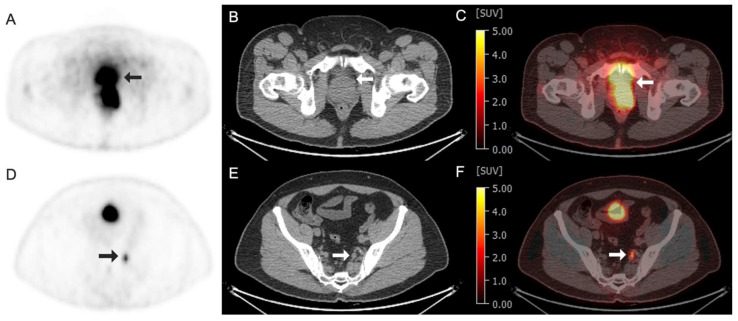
Images obtained from a 60-year-old man with prostate cancer at the level of prostate (A–C) and internal iliac node (D–F). (A–C) Arrows demonstrated an intense radiotracer uptake at the left aspect of the prostate, representing the malignancy. (A) Gallium 68 (^68^Ga)-labeled RM2 axial PET image; (B) axial CT image; (C) axial fused ^68^Ga–RM2 PET/CT image with an SUV max of 24. (D–F) Arrows demonstrated a radiotracer avid in the sub-centimeter left internal iliac node, subsequently biopsied, and consistent with metastasis. (D) ^68^Ga–RM2 axial PET image; (E) axial CT image; (F) axial fused ^68^Ga–RM2 PET/CT image with an SUV max of 8.4.
